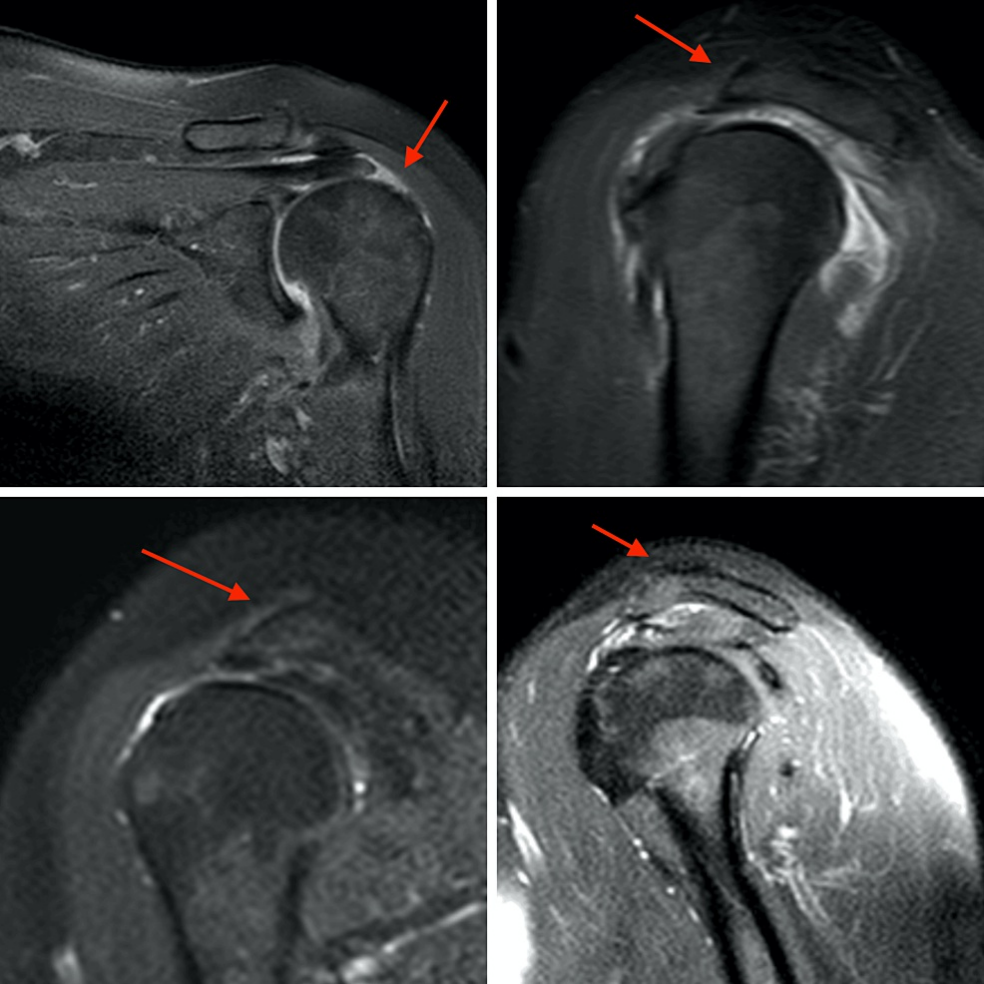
Magnetic resonance images of RCT and type 3 acromion. RCT: Rotator cuff tear
